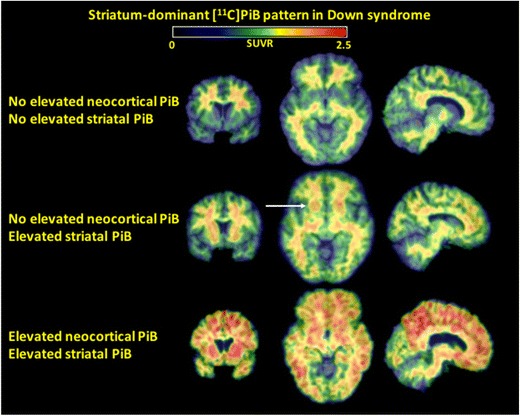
Three representative [^11^C]PiB standard uptake value ratio (SUVR) images. Each row is a representative subject, with the middle row demonstrating the striatum-dominant patterns
